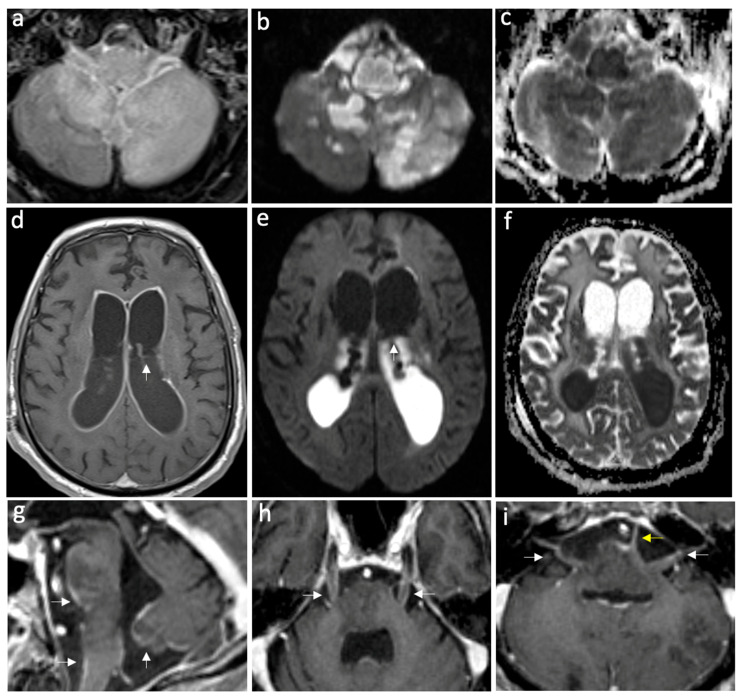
Case of complicated meningoencephalitis with ventriculitis and empyema secondary to *Salmonella enterica*. Axial FLAIR post-gad (a), DWI, and ADC map (b,c) show abnormal parenchymal hyperintensities and diffusion restriction in the medulla oblongata and bilateral cerebellar hemispheres, consistent with encephalitis. Furthermore, note the restricted diffusion in the prepontine cistern, consistent with empyema. Axial T1 post-gadolinium (d), DWI, and ADC map (e,f) show intense ventricular ependymal surface enhancement related to ventriculitis and intraventricular diffusion restriction due to intra-ventricular empyema, with a pus fluid level indicated by white arrows. On the sagittal post-gadolinium image (g), leptomeningeal enhancement is visible on the ventral pons, medulla oblongata, and inferior vermis, indicating meningitis (white arrows). The axial post-gadolinium images show leptomeningeal enhancement along the bilateral trigeminal nerves (h), bilateral facial and vestibulocochlear nerves (white arrows), and the left abducent nerve (yellow arrow) (i).
